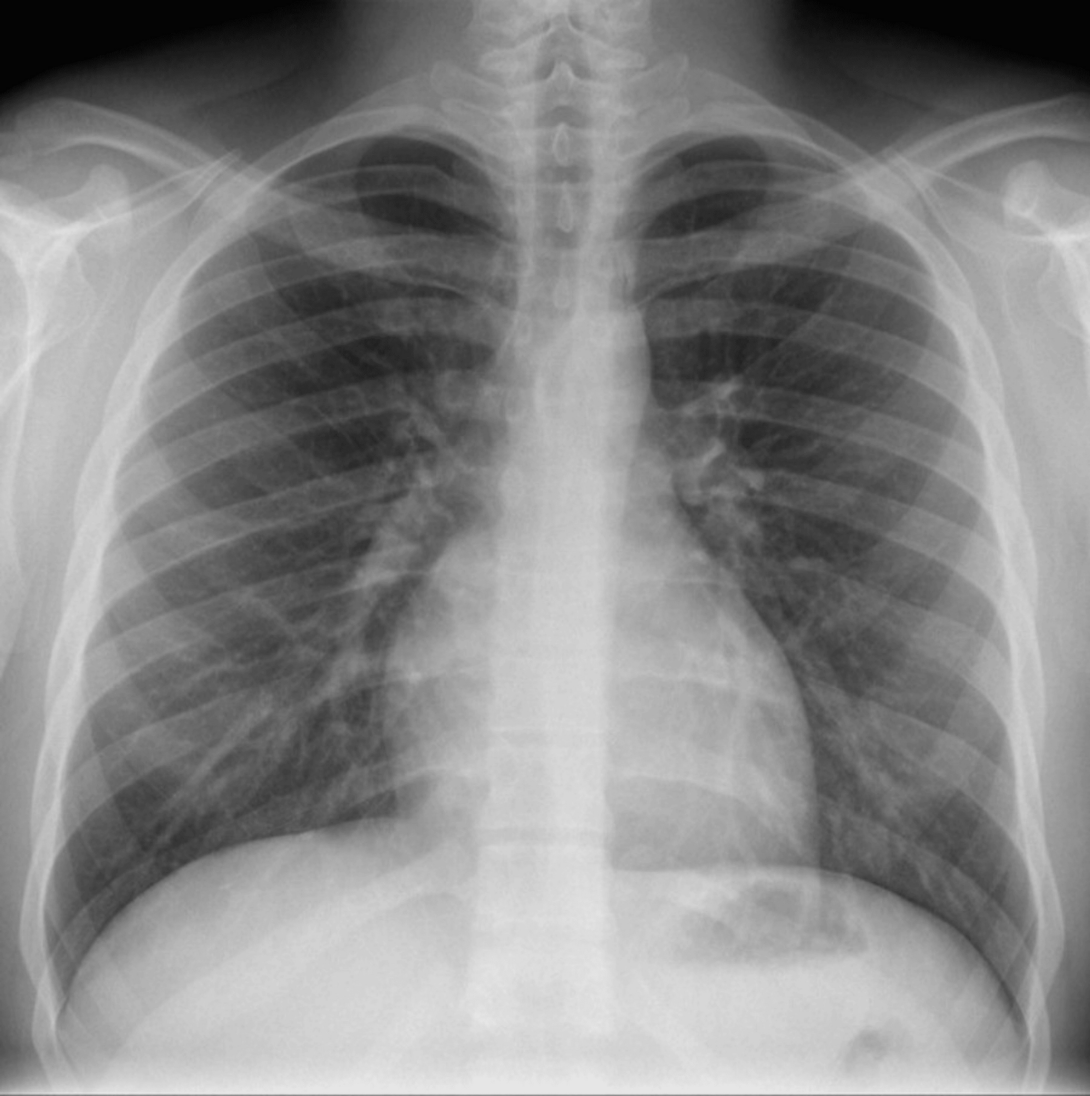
Chest X-ray of the patient.
